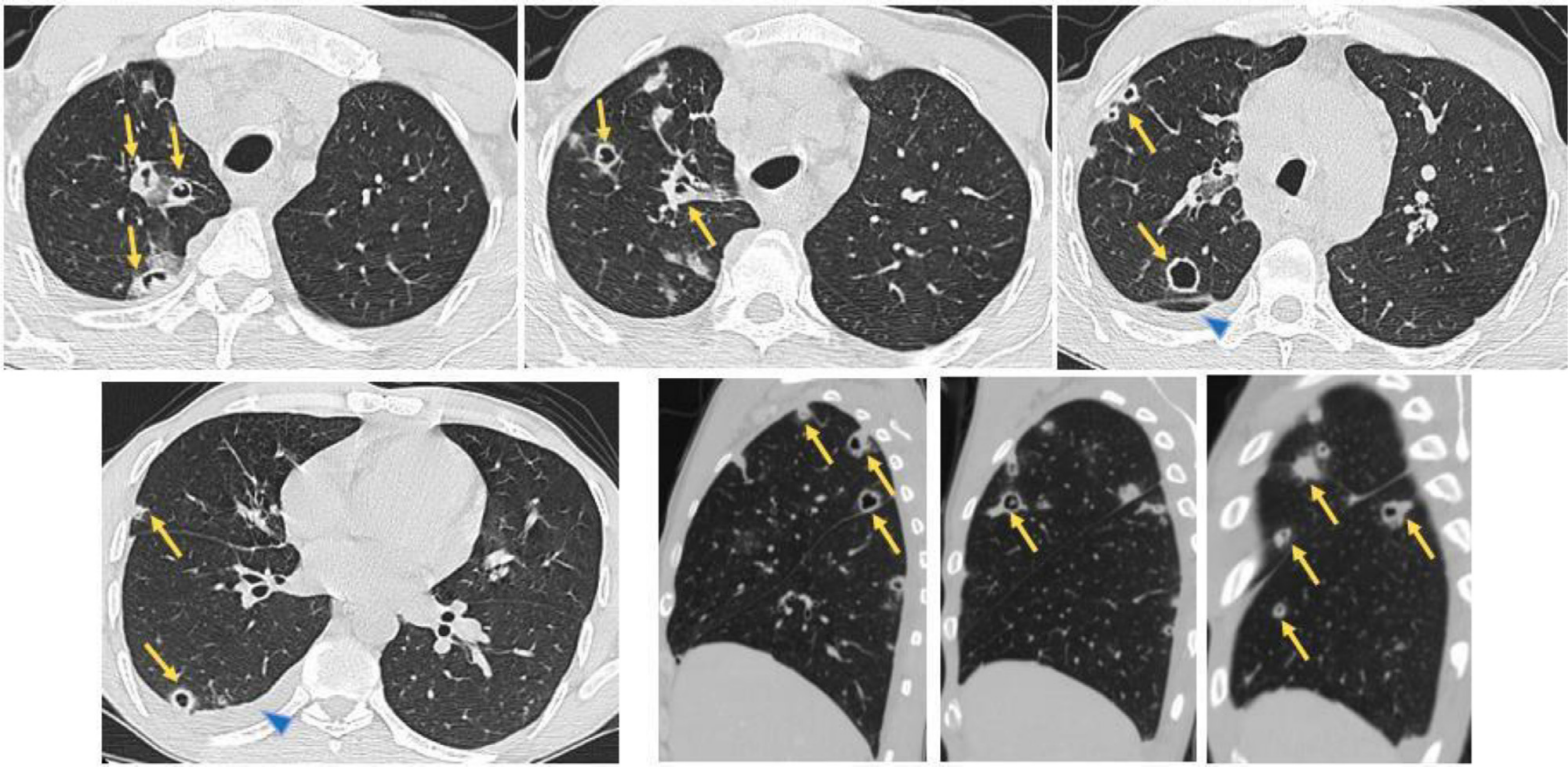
Axial contrast-enhanced CT images (lung window) show multiple right unilateral pulmonary nodules, of random distribution, of different shapes and sizes, excavated for the vast majority (Arrows), with a low abundance of right pleural effusion (Arrowhead).
